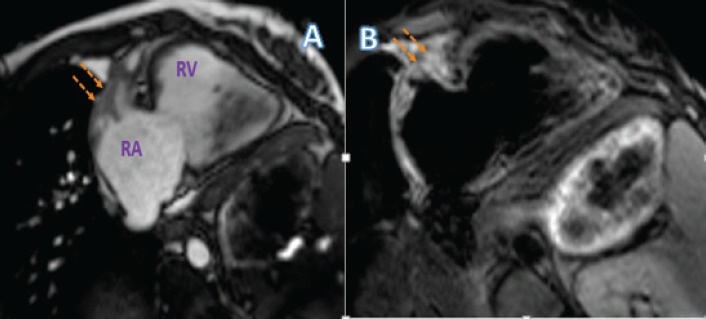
(a) Cardiac MRI of the patient post two cycles of chemotherapy with cine RV-2 chamber view showing the reduced pericardial mass encasing the right AV groove. (b) Tissue characteristics of the right atrial mass.
